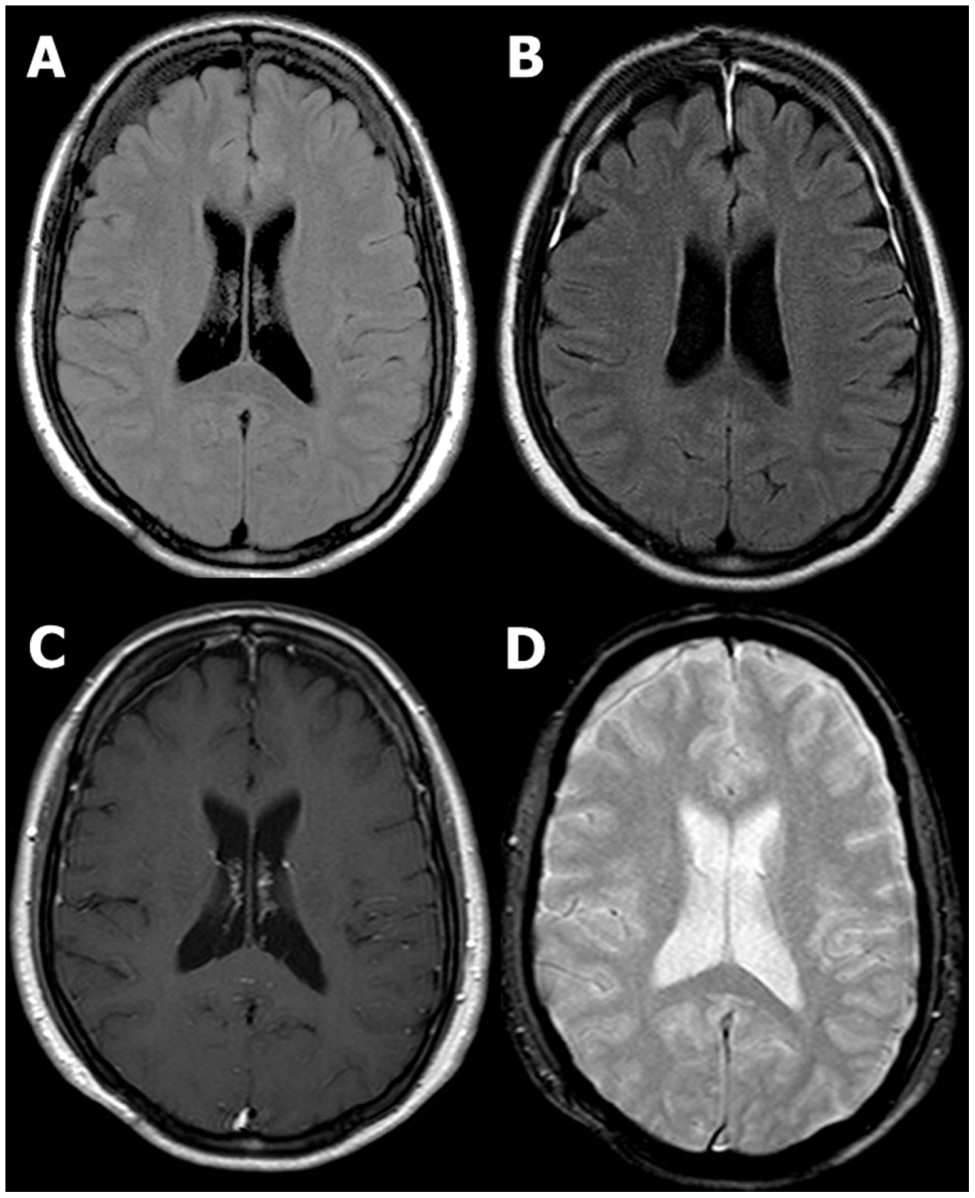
A 60-year- old female patient with a history of assault 10 hours ago. Initial GCS score was 15. The duration of posttraumatic amnesia was 3(B) Contrast-enhanced FLAIR MR image helped to detect small amount of subdural hemorrhage in both frontal convexity which was initially missed, after reviewing meningeal enhancement. No demonstrable abnormality was found on unenhanced FLAIR (A), contrast-enhanced T1 weighted (C) and GRE (D) MR images.
